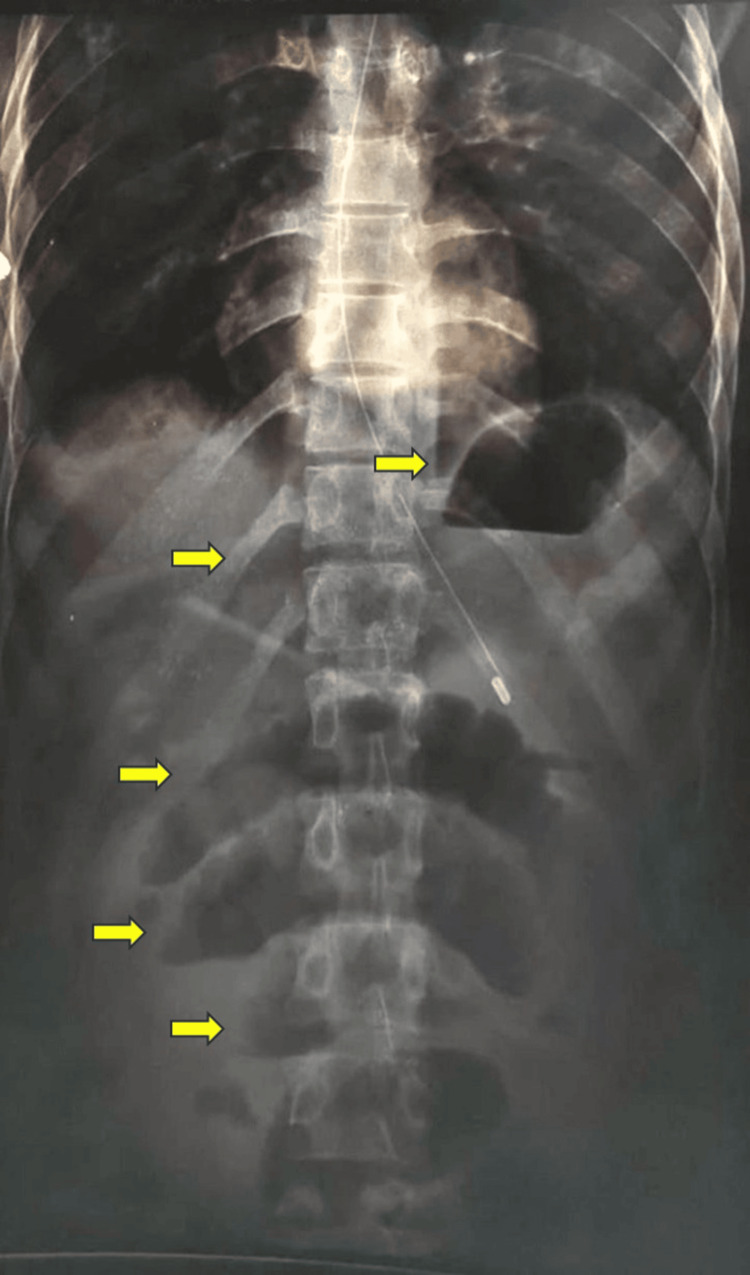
X-ray of the erect abdomen upon the arrival of the patient at the hospital The yellow arrows demonstrate multiple air-fluid levels.
